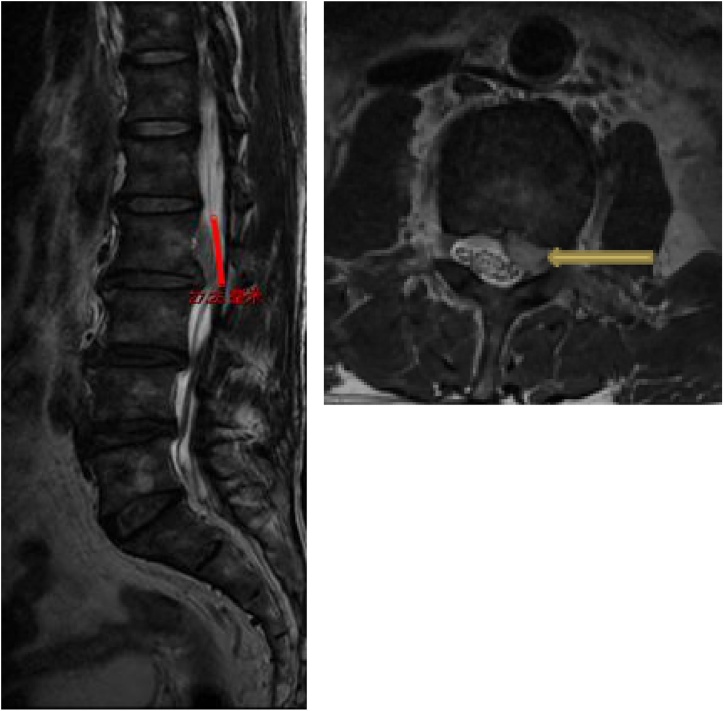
MRI showed that a large disc fragments extruded upward into L2-3 intervertebral space and the left nerve root was compressed.
